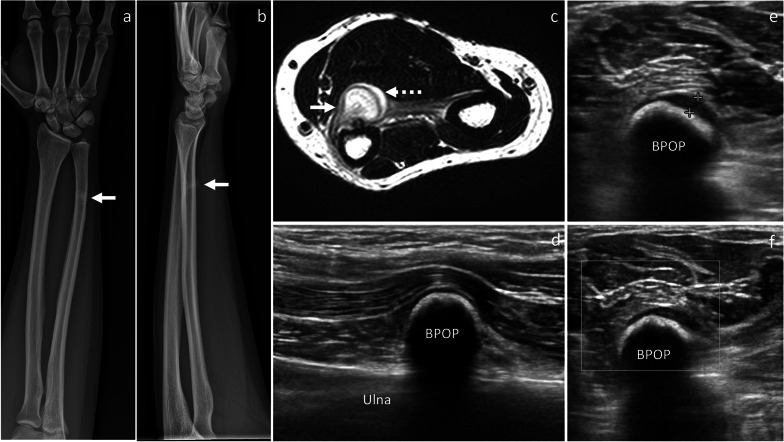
BPOP arising from the distal ulnar diaphysis. On X-rays, frontal (a) and lateral (b) views show a mineralized mass, which is contiguous to the distal diaphysis of the ulna. On axial T2-weighted MRI sequence (c), the mass is hyperintense with higher signal at the periphery representing cartilage covering (dashed arrow). Longitudinal B-mode ultrasound image (d) depicts a calcified mass, which is contiguous to the palmar aspect of the ulna and impinges on the flexor muscles. Axial B-mode ultrasound image (e) shows a thin hypoechoic layer (calipers) superficial to the mineralized mass, which is in keeping with cartilage covering. No increased vascularity is seen on power Doppler imaging (f). After biopsy, surgical resection was performed and BPOP was pathologically proven. White arrow points at BPOP in all images
